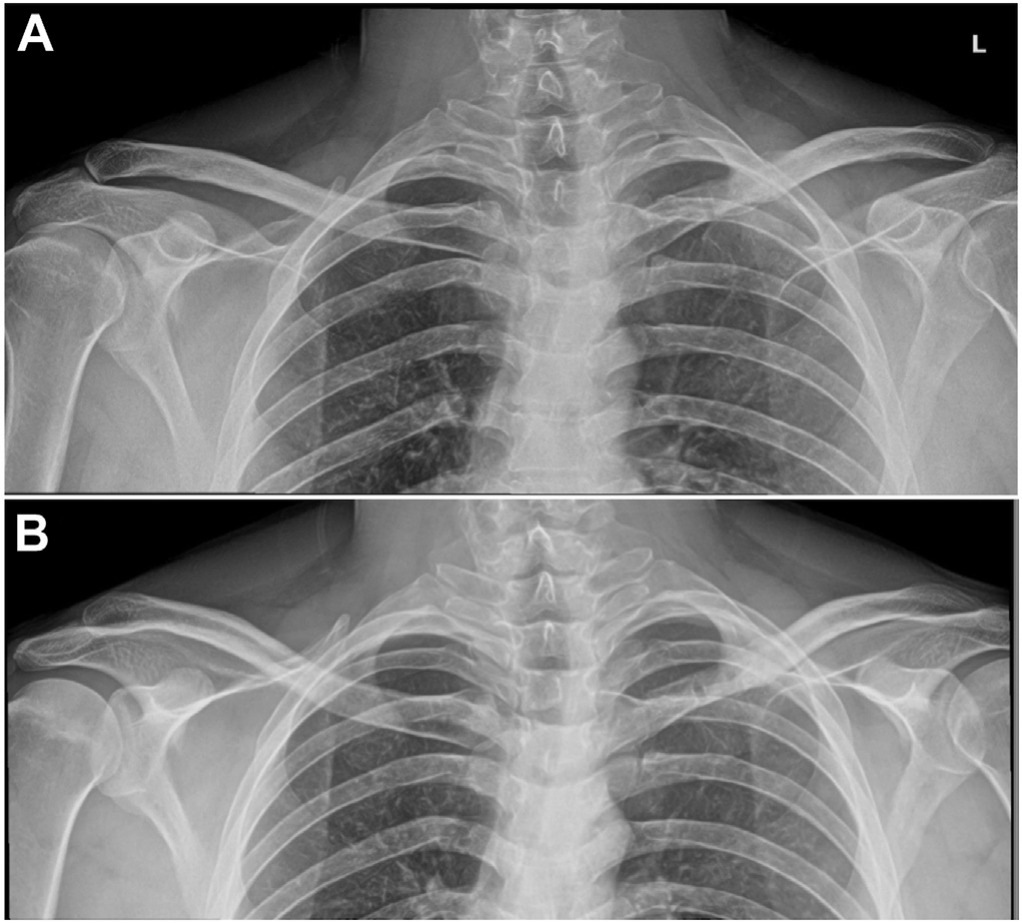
Two postoperative radiographic images, including (A) anteroposterior view of the clavicle with cephalic angulation and (B) anteroposterior view of the left clavicle, after hardware removal show a healed fracture.
